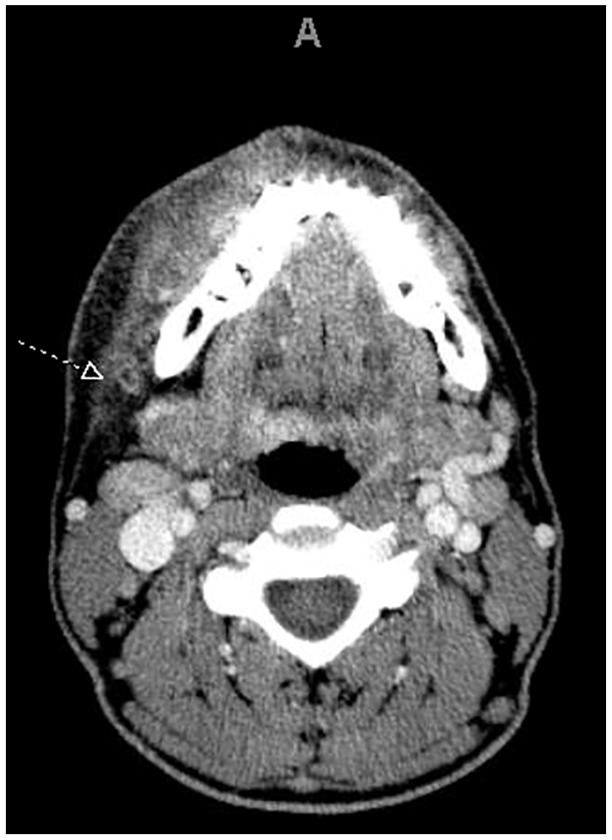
Contrast-enhanced computed tomography scan of neck showing right facial vein thrombosis (arrow) with surrounding inflammation.
